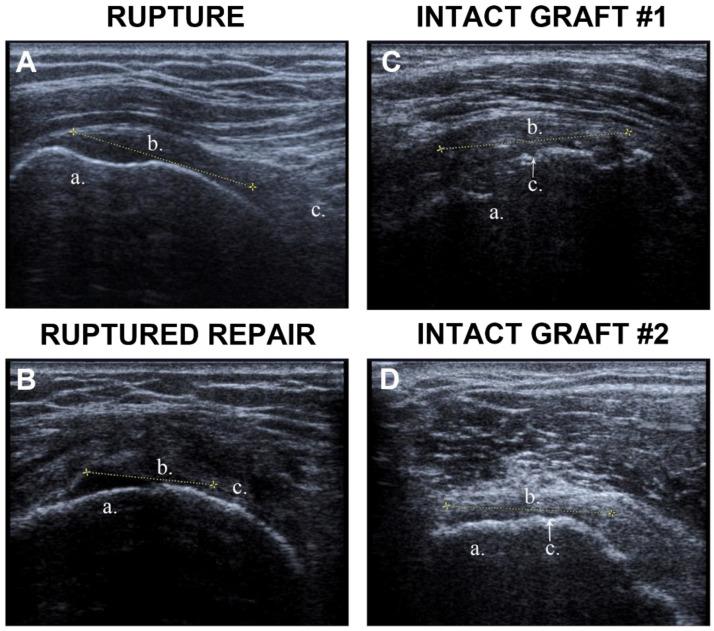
Ultrasound image of the rotator cuff tears and repairs from representative patients. (A) Rotator cuff tear. a. humeral head; b. defect; c. retracted cuff. (B) Ruptured repair. a. humeral head; b. defect; c. retracted cuff and graft. (C,D) Intact GraftJacket^®^ and cuff repair. a. humeral head; b. intact graft; c. suture. Adapted with permission from [91].
